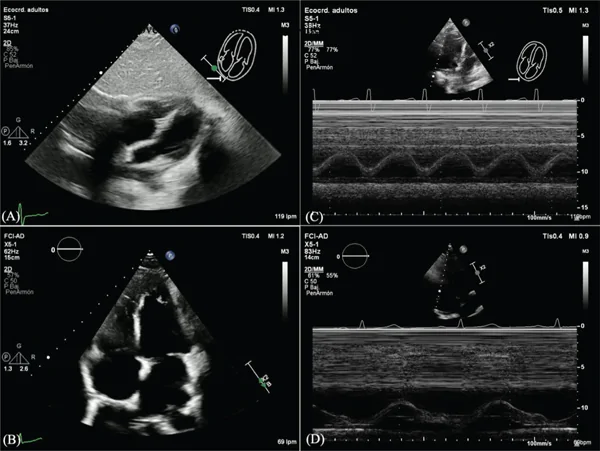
Comparative echocardiographic findings between admission and 3‐month follow‐up. (A) Apical four‐chamber view on admission showing right ventricular dilation and septal flattening consistent with pressure overload. (B) Follow‐up apical four‐chamber view showing normalization of right ventricular size and septal position. (C) TAPSE measurement at admission showing reduced longitudinal right ventricular function (12 mm). (D) Follow‐up TAPSE demonstrating recovery of right ventricular systolic function (27.7 mm).
